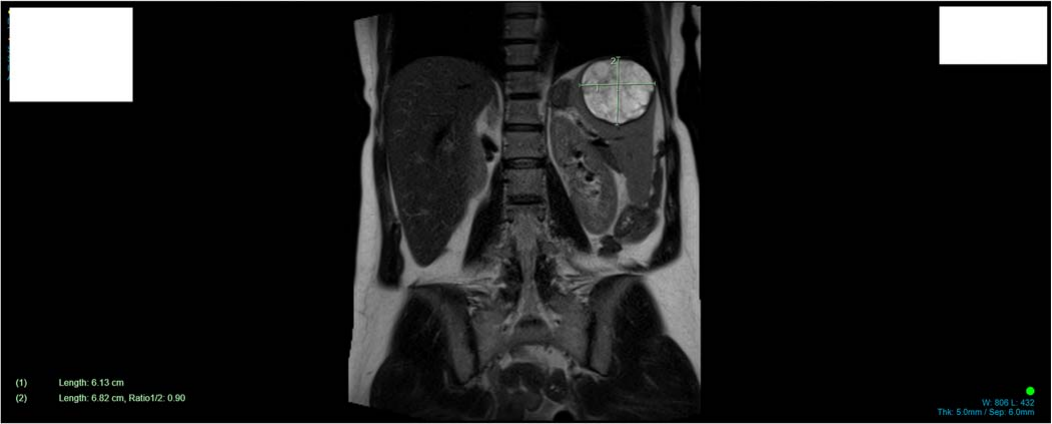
MRI demonstrating size and anatomical location of splenic lesion coronal plane.
